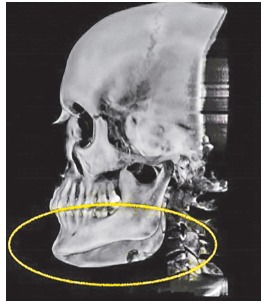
Computed tomography in lateral view evinces asymmetry between mandibular bodies.
